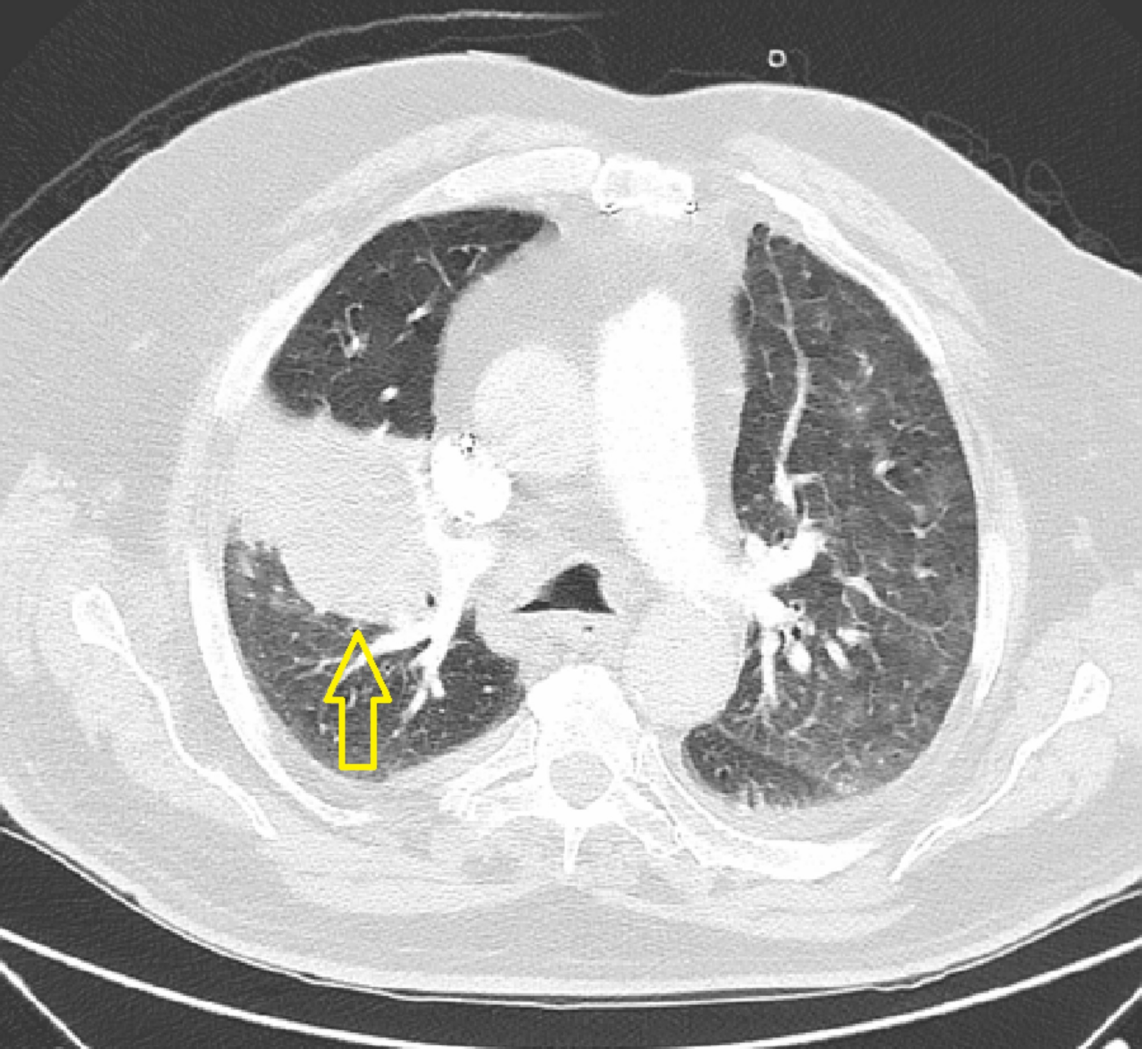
Transverse View of Computed Tomography Chest
